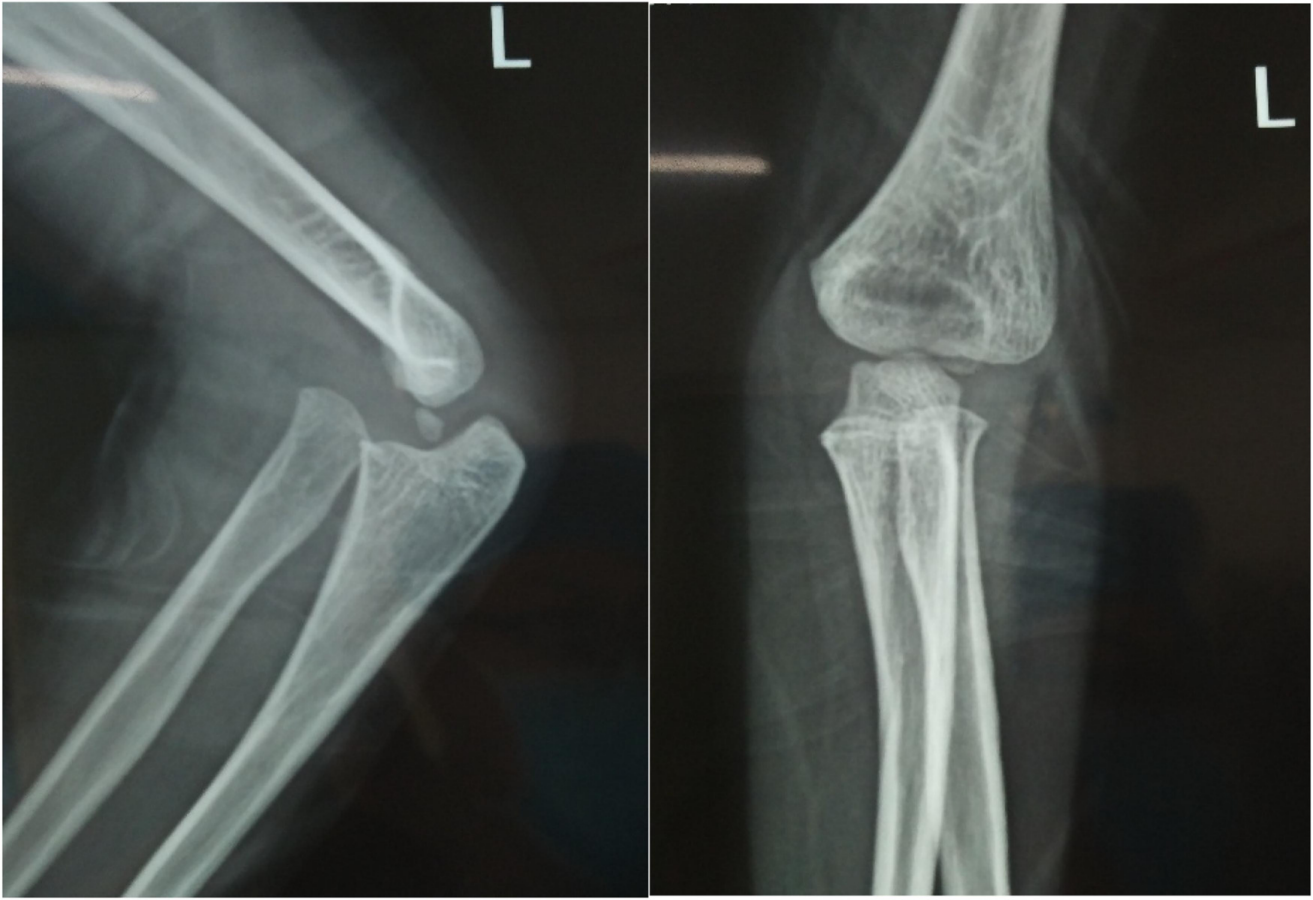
The x-ray demonstrated the anterior dislocation of the radial head.
